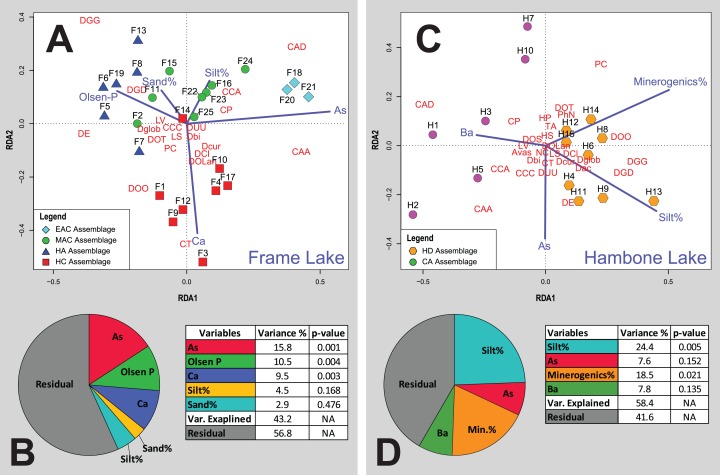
Redundancy analysis (RDA) tri-plots and variance partitioning results. (A) RDA triplot for the Frame Lake datasets. (B) Variance partitioning of the partial RDA of the Frame Lake dataset. (C) RDA triplot for the Hambone Lake datasets. (D) Variance partitioning of the partial RDA of the Hambone Lake dataset. The colored symbols represent the samples of the identified assemblages in Frame Lake and Hambone Lake. The dark blue lines represent the variables selected in both lakes. CCA, *Centropyxis aculeata* “aculeata”; CCD, *Centropyxis aculeata* “discoides”; CCA, *Centropyxis constricta* “aerophila”; CCC, *Centropyxis constricta* “constricta”; CT, *Cucurbitella tricuspis*; CP, *Conicocassis pontigulasiformis*; HP, *Hyalosphenia papilio*; HS, *Heleopera* sphagni; LV *Lagenodifflugia vas*; LS *Lesquereusia spiralis*; PC *Pontigulasia compressa*; PhN, *Phryganella nidulus*; DGG, *Difflugia glans* “glans”; DGD, *Difflugia glans* “distenda”; Dglob *Difflugia globulosa*; DUU, *Difflugia urceolata* “urceolata”; DOO, *Difflugia oblonga* “oblonga”; DOT, *Difflugia oblonga* “tenuis”; DOLan, *Difflugia oblonga* “lanceolata”; DE, *Difflugia elegans*; Dbi, *Difflugia bicornis*; DA, *Difflugia protaeiformis* “acuminata”; DCl, *Difflugia protaeiformis* “claviformis”; DCur, *Difflugia curvicaulis*.
